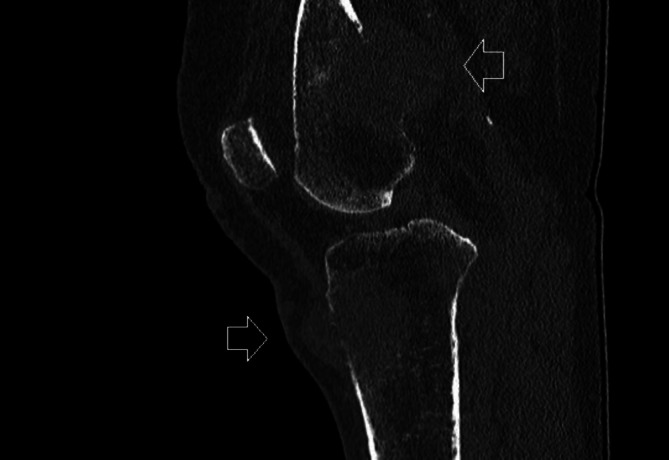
Preoperative radiograph of the knee (lateral view) demonstrating lytic metastatic lesions of both the proximal tibia and the distal femur with cortical destruction.
